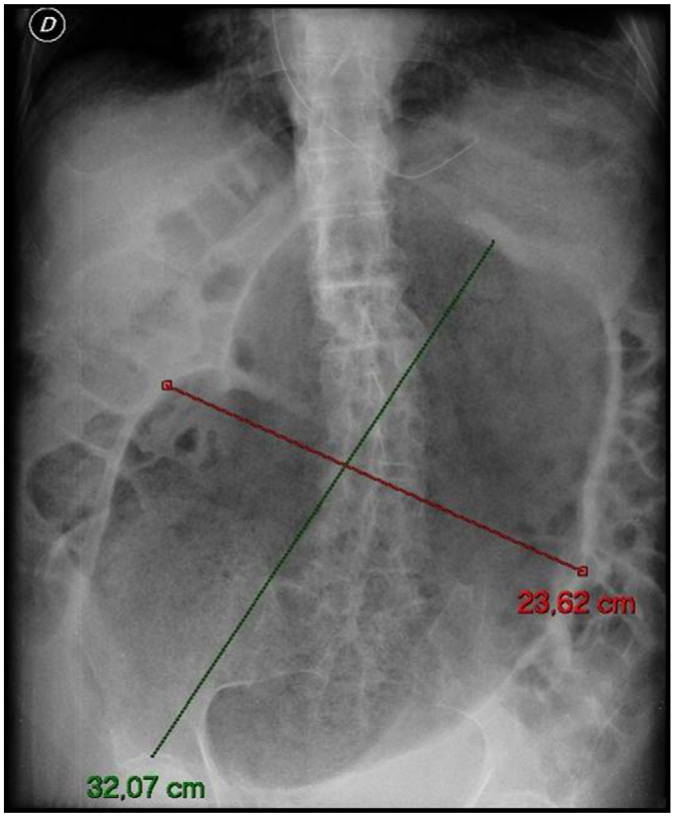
Plain abdominal X-ray after two days of medical treatment (rehydration, correction of electrolyte imbalance, and multiple enemas) showing enormous faecal impaction, although slightly reduced, still measuring 32 cm in length and 24 cm in width.
